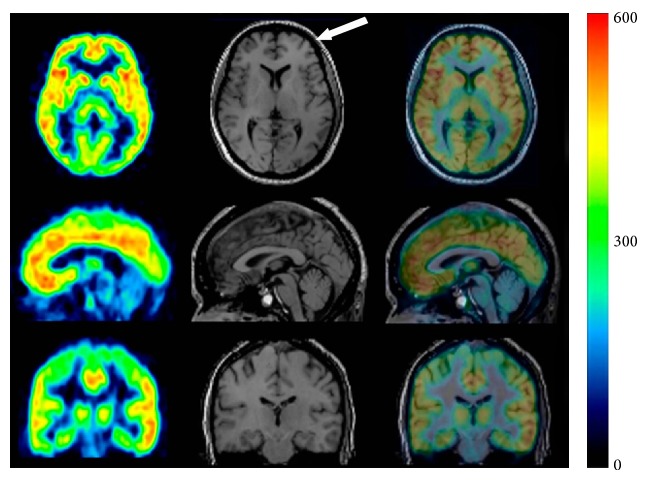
PET/MR images of [^18^F]SP203 (60–180 min) in healthy subject. Arrow points to subcutaneous fat [101].
